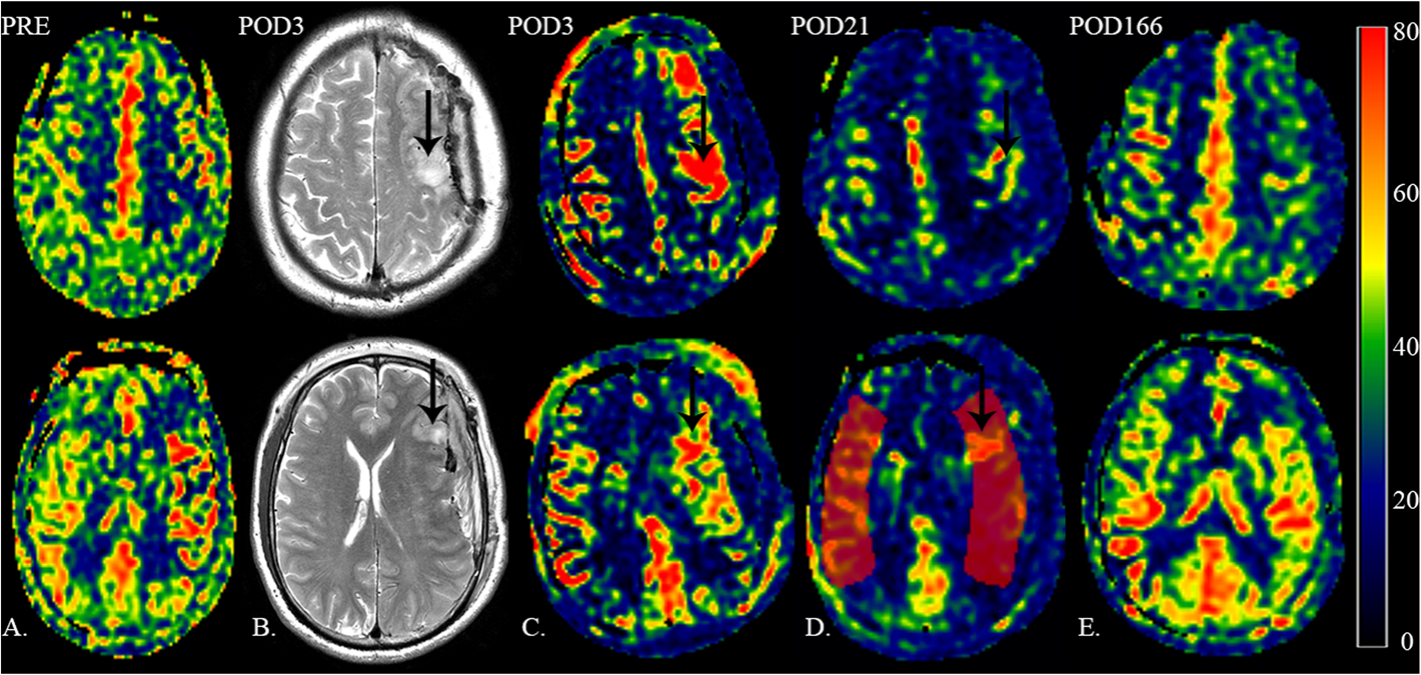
The changes of cerebral blood flow. a. Left cerebral perfusion is lower than right cerebral perfusion on preoperative magnetic resonance imaging 3D–arterial spin labeling (MRI 3D-ASL). b. T2-weighted magnetic resonance imaging showed mild focal cerebral edema in the operative area and a swollen temporal muscle on the 3rd day after surgery (The black arrow indicates cerebral edema at the sites of anastomosis). c. MRI 3D-ASL showed increased cerebral blood flow (CBF) at the sites of anastomosis with mildly increased CBF of the adjacent cerebral cortex on the 3rd day after surgery (The black arrow indicates increased CBF at the sites of anastomosis). d. MRI 3D-ASL shows high CBFs in the region distributed by dual recipient vessels compared with the adjacent cerebral cortex, while cerebral perfusion of the whole brain displays a relative low level on the 21st day after surgery (The black arrow indicates high CBFs, and the red circle indicates the region of interest of the middle cerebral artery (MCA) distribution). e. MRI 3D-ASL examined at 6 months after surgery shows good improvement on the left side and a normal level on the right side
